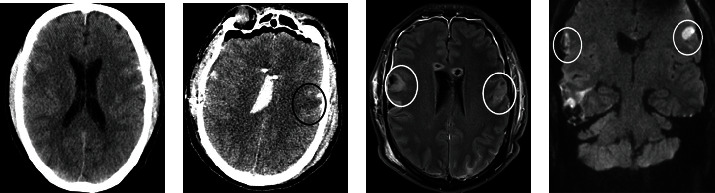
(a) Initial CT scan showing acute traumatic changes. (b) The postoperative CT revealed a subacute ischaemic lesion on the left operculum (black circle) and residual post-traumatic and postoperative changes. On the T2-weighted MR images (3T Magnetom Vida, Siemens, Erlangen, Germany), (c) hyperintense lesions in both opercula (white circles) are detected which are due to parenchymal defect caused by contusion on the right side and due to an ischaemic event on the left side, as clearly seen in the (d) diffusion-weighted images (DWI).
